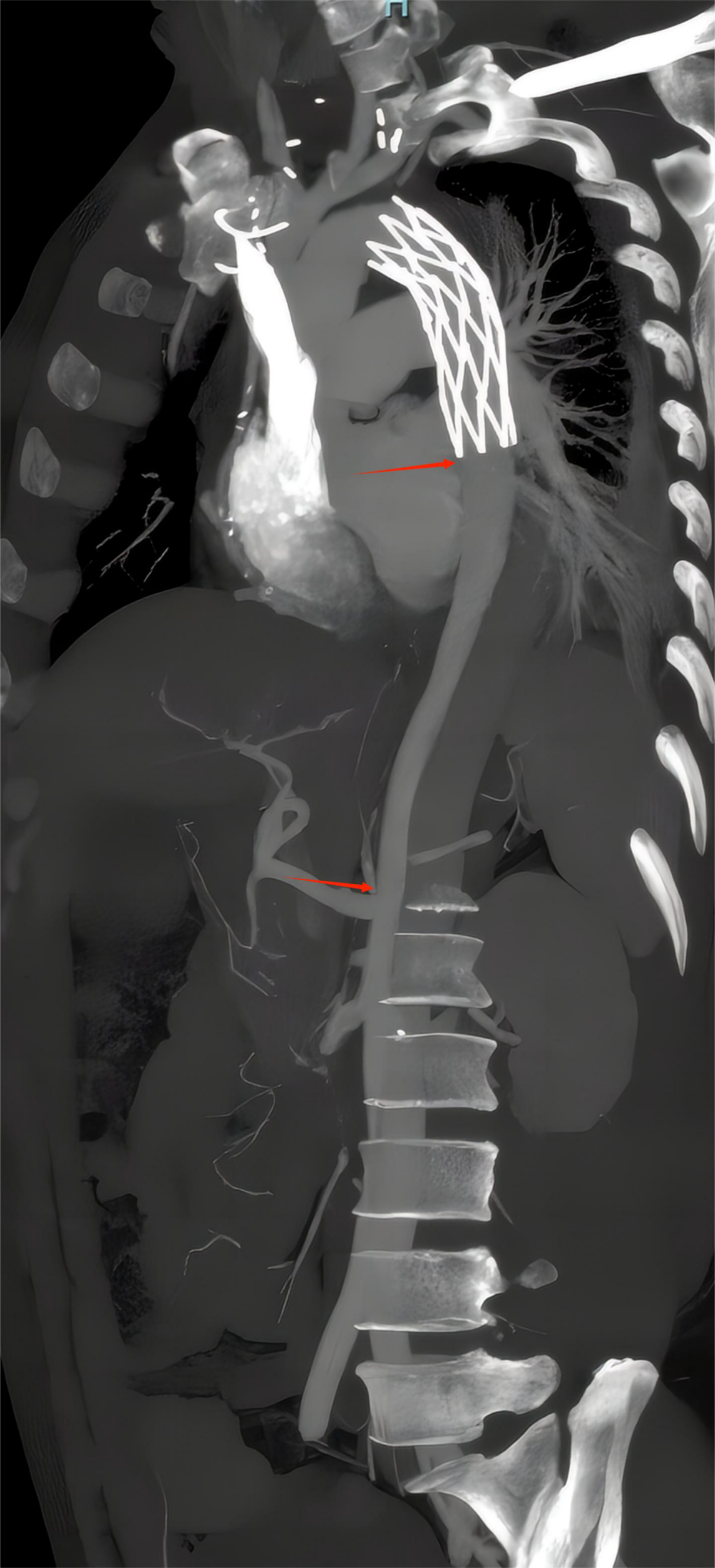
Zoning of CTA. The s1 plane and s2 plane in the longitudinal section.
